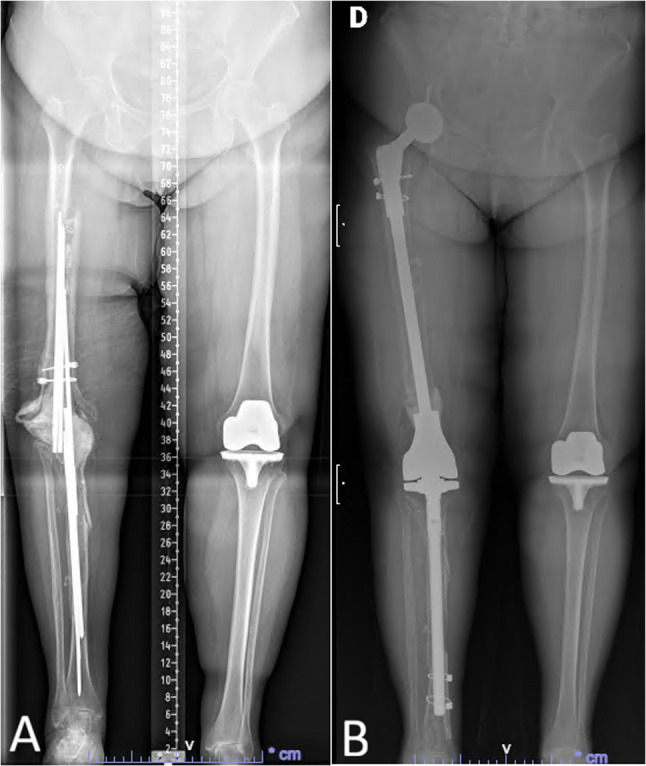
A Preoperative anteroposterior radiograph of a woman with knee osteoarthritis complicated by chronic infection, operated on several times, with a fracture of the femur and tibia during the last surgery. B Radiograph at three-year follow-up after reconstruction with a total femoral replacement using a push-through stem
